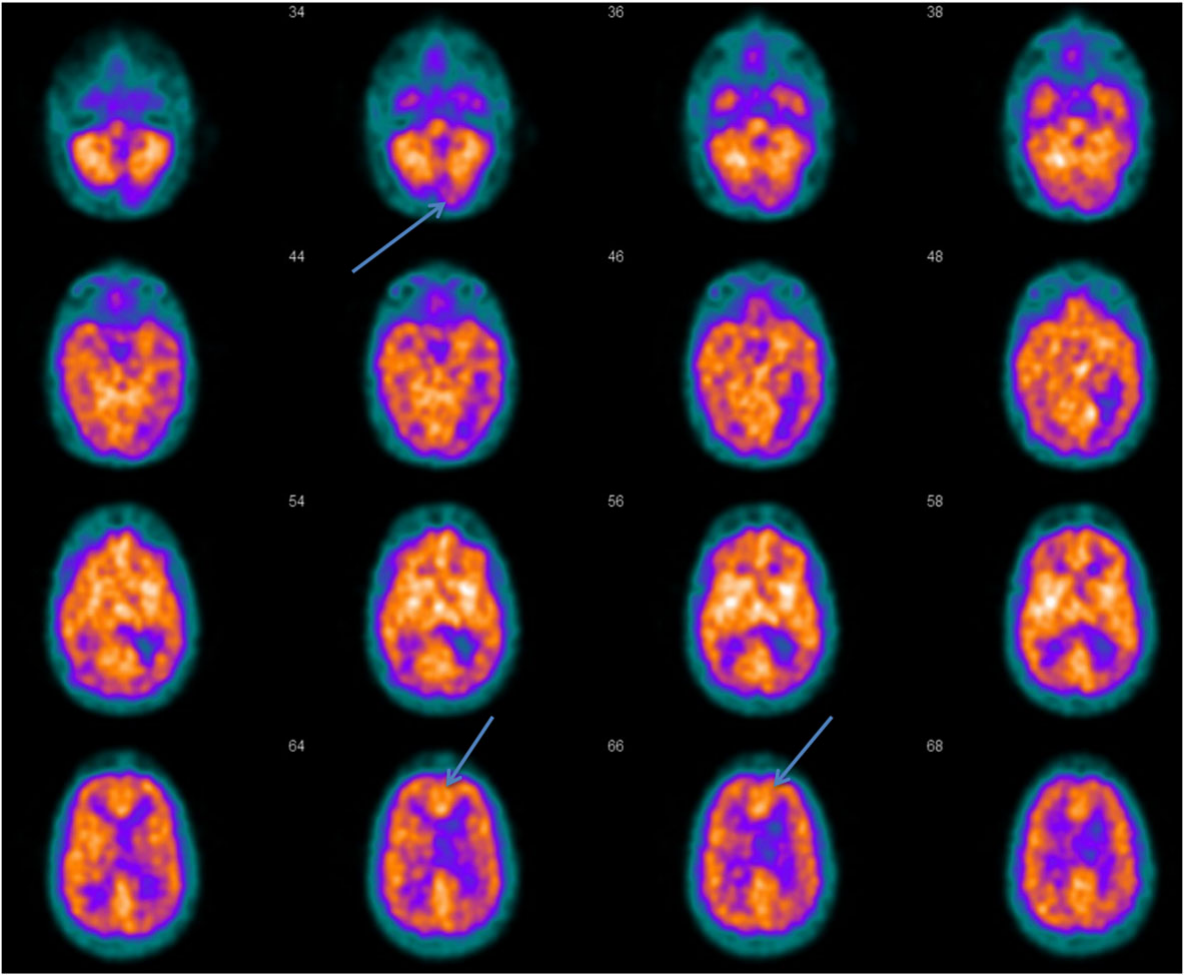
99 m Tc- HMPAO Perfusion SPECT scan showing focally increased perfusion at the level of the anterior cingulate (visualized on the last row, blue arrow). The blue arrow in the first row shows absent perfusion in the posterior fossa, as expected post-surgical finding
